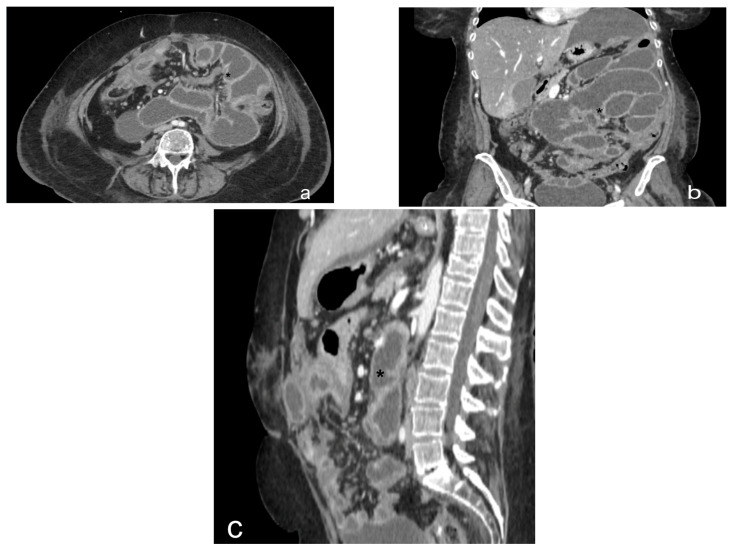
Contrast-enhanced CT scan, axial view (a), coronal view (b), sagittal view (c). Case of ovarian carcinoma in a female, 50 y. Peritoneal carcinosis nodules (black star) involving fascia trasversalis and the ileal loops in the right flank, causing fluid distension of the proximal loops.
